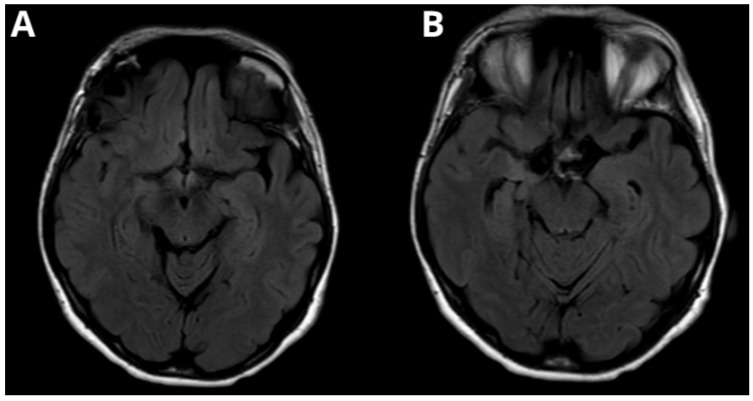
Postoperative FLAIR MRI of the patient’s dysembryoplastic neuroepithelial tumor (DNET) in the right temporal lobe. (A) Axial scan showing no residual tumor and stability of intracranial anatomical structures following surgical intervention. (B) Axial scan at a more caudal level to scan B, confirming complete resection and showing the resection cavity.
